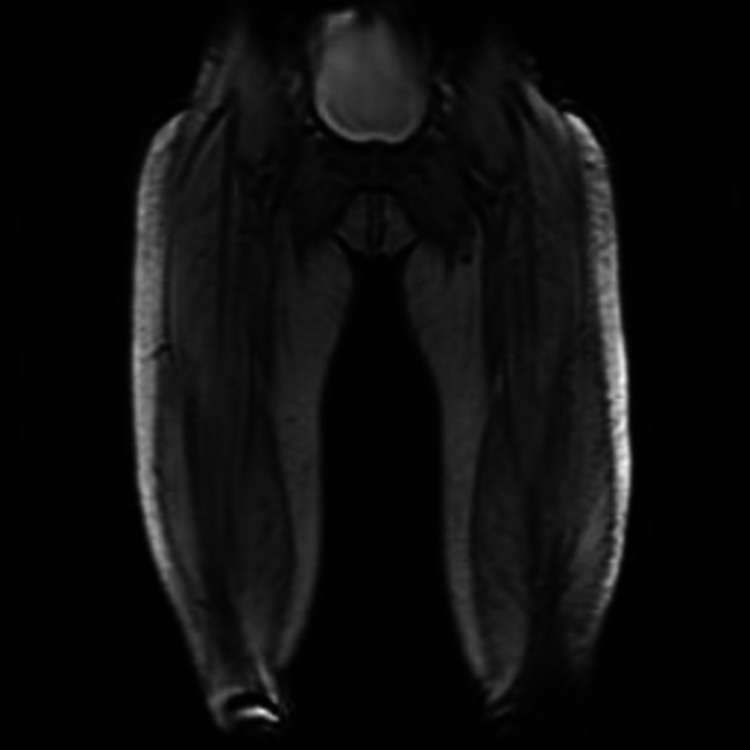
MRI scan lower limb showed proximal muscle myositis
